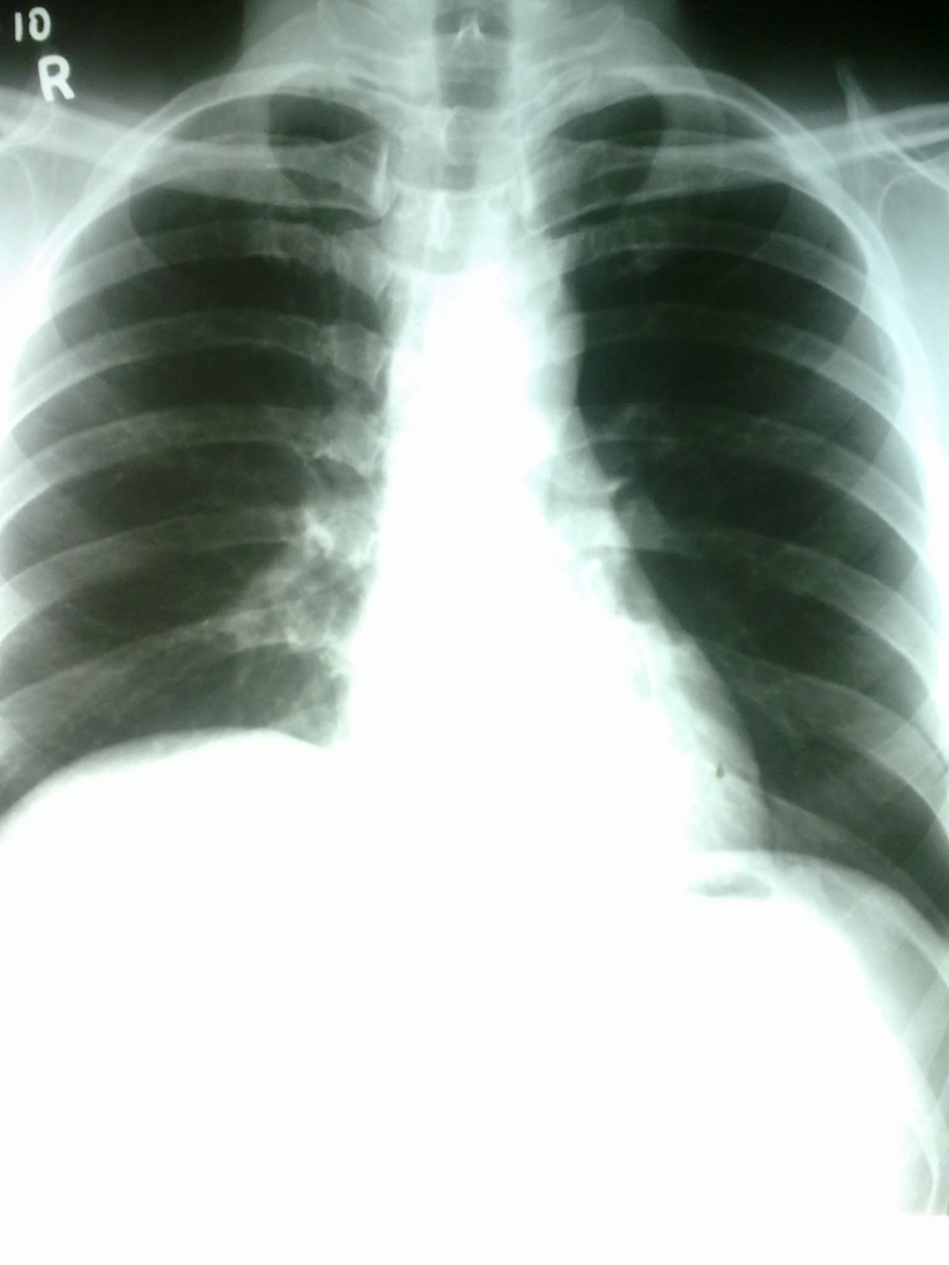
Chest X-Ray on admission.
